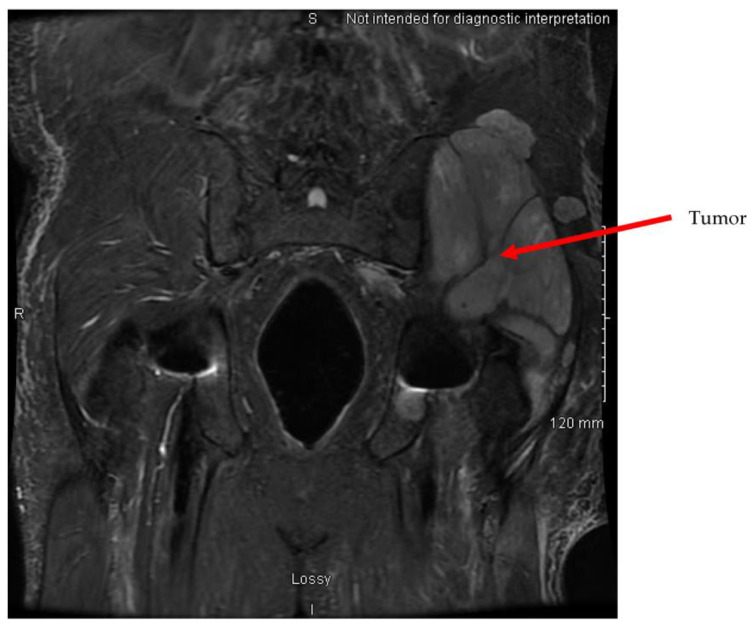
MRI abdomen pelvis, extensive tumor in left hip appears bright on T2 coronal MRI.
